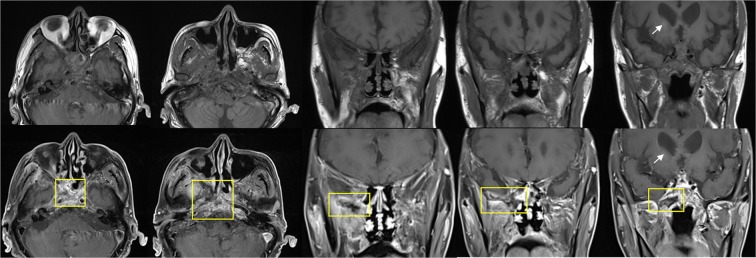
Preoperative MR images of a 69-year-old male patient who had extensive extrasinonasal acute invasive fungal sinusitis lesions and died of acute invasive fungal sinusitis progression. T1-weighted images (upper) and gadolinium-enhanced T1-weighted images (lower) show that right middle cranial fossa skull base, zygoma, maxillary sinus, pterygopalatine fossa, infratemporal fossa, and cavernous sinus were involved with mucormycosis. loss of contrast enhancement lesions reflecting a necrosis (yellow square) were observed in the right greater wing of sphenoid bone, sphenoid body, right pterygopalatine fossa, right infratemporal fossa, right pterygoid plate, right zygoma, and right maxillary sinus posterior wall. Soft tissue inflammation was identified in the right orbital apex, right cavernous sinus with internal carotid artery narrowing, both prevertebral space, right parapharyngeal space, and masticator space. Acute infarction in the right basal ganglia (white arrow) and enhancement of meninx were also found. Finally, he died of infarction of right anterior cerebral artery territory due to meningoencephalitis and skull base osteomyelitis.
